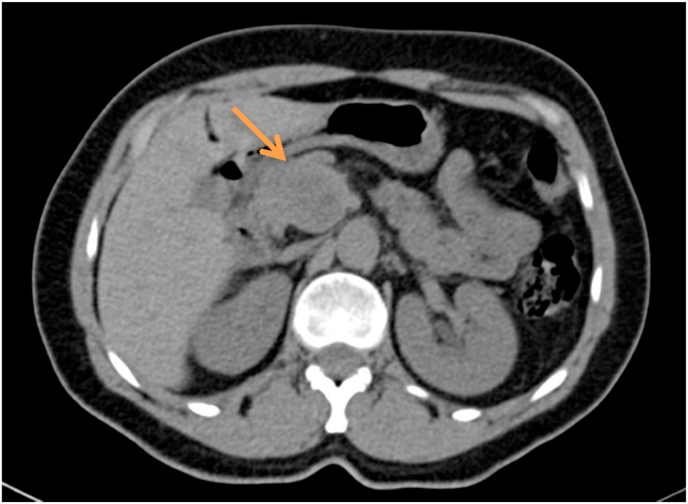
Spiral CT of the abdomen before contrast administration reveals a 35mm hypodense lesion with well-defined borders in the pancreas's head (arrow).
